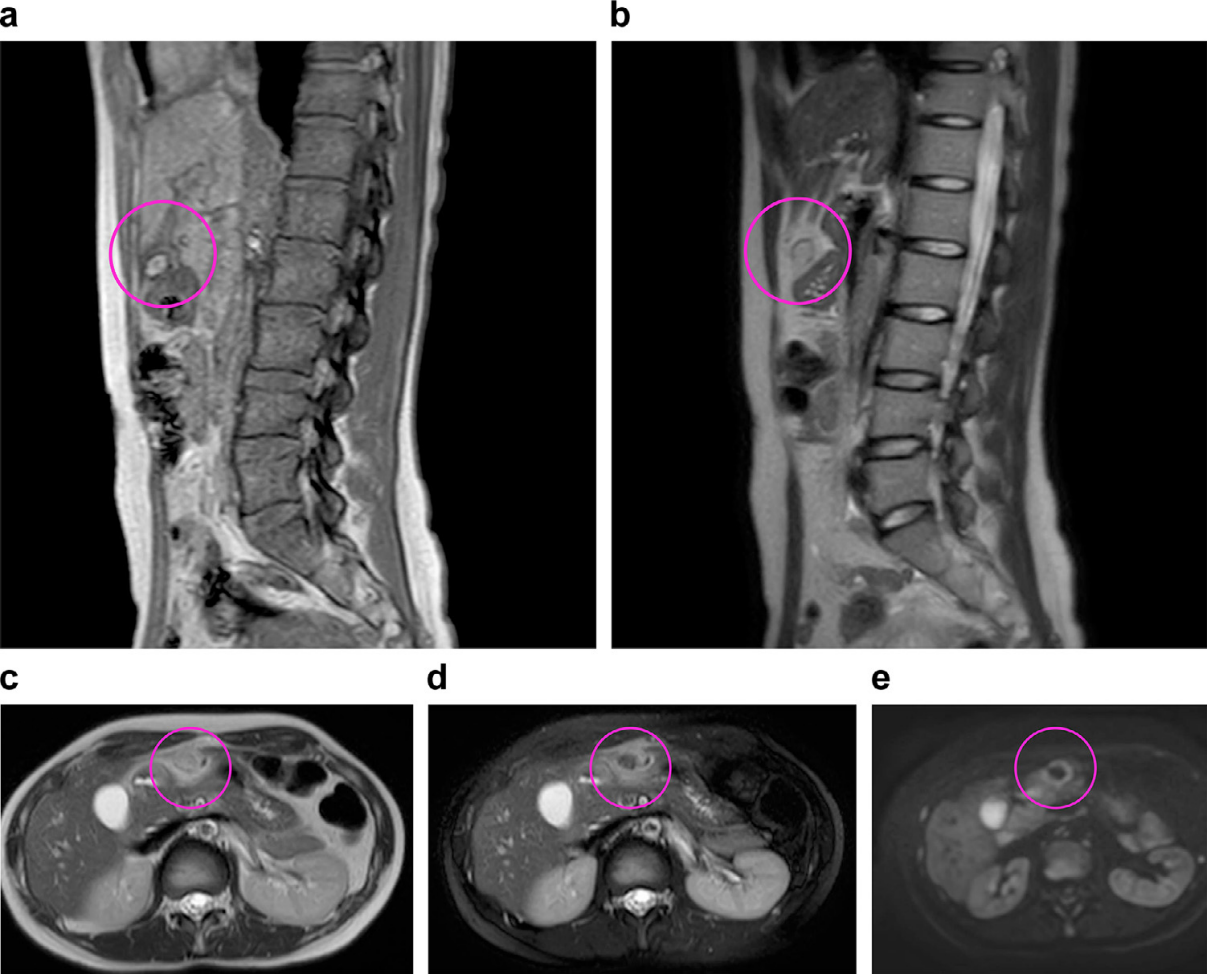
Magnetic resonance imaging findings in Case 2. (A) T1-weighted imaging, (B) T2-weighted imaging, (C) T2-weighted imaging, (D) fat-suppressed T2-weighted imaging, (E) Diffusion-weighted imaging. T1-weighted imaging shows a 1-cm oval mass with high signal on the right side of the hepatic round ligament (A) (circle). The mass is comparable with the surrounding fat on T2-weighted imaging (B, C) and is hypointense with surrounding high signal area on fat-suppressed T2-weighted imaging (D). Diffusion-weighted imaging shows high signal only on the margins of the mass (E).
